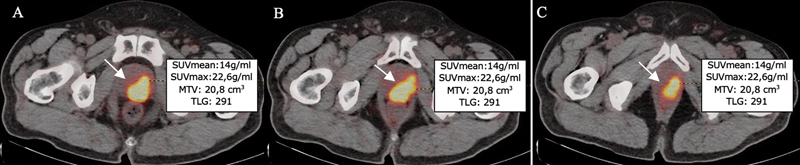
Pretreatment gallium-68-labeled prostate-specific membrane antigen (Ga-68-PSMA) positron emission tomography/computed tomography (PET/CT) images of a 59-year-old prostate cancer (PCa) patient categorized as Gleason score (GS): 4 + 5, D'Amico high risk, high-risk Candiolo (HRC), metastatic tumor. Summary of maximal standardized uptake value (SUVmax), mean SUV (SUVmean), metabolic tumor volume (MTV), and total lesion glycolysis (TLG) values derived from primary prostate tumor (arrow) on axial fused pretreatment 68Ga-PSMA PET/CT images of a patient with low risk (
